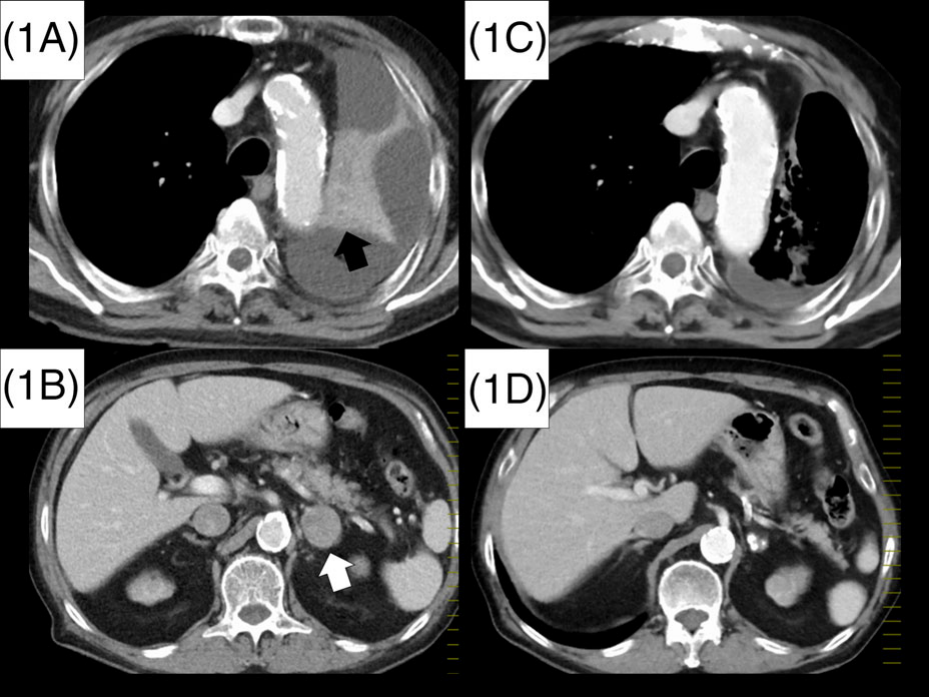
Following one course of amrubicin, a CT scan revealed an enlargement of the locally recurrent pulmonary nodule (black arrow in Figure 1A), peripheral atelectasis, and an increased size of the left adrenal lesion (white arrow in Figure 1B), indicating progressive disease. Subsequently, the administration of carboplatin, etoposide and atezolizumab was initiated. Although due to adverse events, carboplatin and etoposide were discontinued after a single course, the patient exhibited a reduction in the pulmonary nodule and adrenal metastasis while receiving atezolizumab, indicating a partial response that has been sustained for a period of 7 months (Figure 1C and D).
